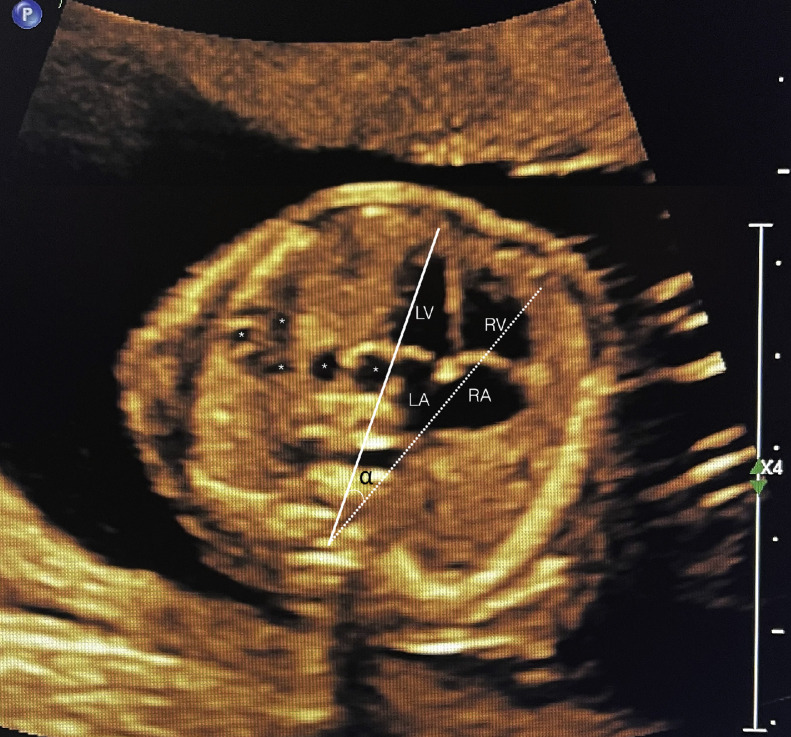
The identical axial sonographic image of the fetal thorax at the level of the four-chamber heart depicted at 21 weeks’ gestation, as in Fig. 2. This figure contains lines defining the degree of displacement of the fetal heart to the right (dextroposition). The midline (solid line) in the normal-positioned heart would have traversed the right cardiac chambers (RA, RV). However, in this dextropositioned fetal heart this line has been considerably displaced to the right, and is represented by the dotted line. The angle between the two lines solid and dotted (marked with a) represents the cardiomediastinal shift angle (CMSA).
